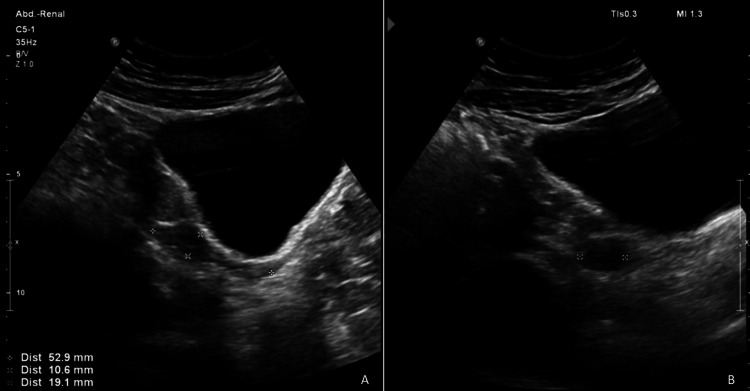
Pelvic Ultrasound (A and B) The uterus is anteflexed, with regular, well-defined contours. The dimensions are 53 mm in the longitudinal axis, 10 mm in the anteroposterior axis, and 19 mm in the transverse axis. The endometrium is central, echogenic, and measures 3 mm.
